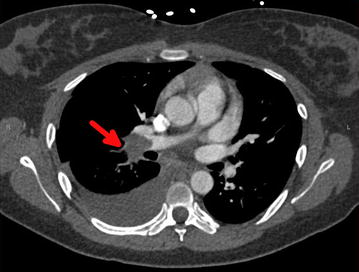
Computed tomography angiography (CTA) of the chest (*transverse view*) demonstrating thrombus in the *right* pulmonary artery (*red arrow*)
